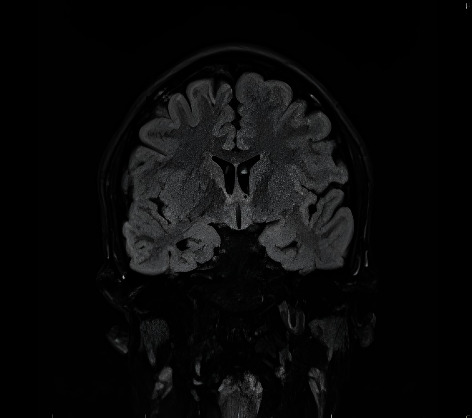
MRI brain coronal view showing generalised atrophy including mild bilateral hippocampal atrophy with no evidence of sclerosis.
